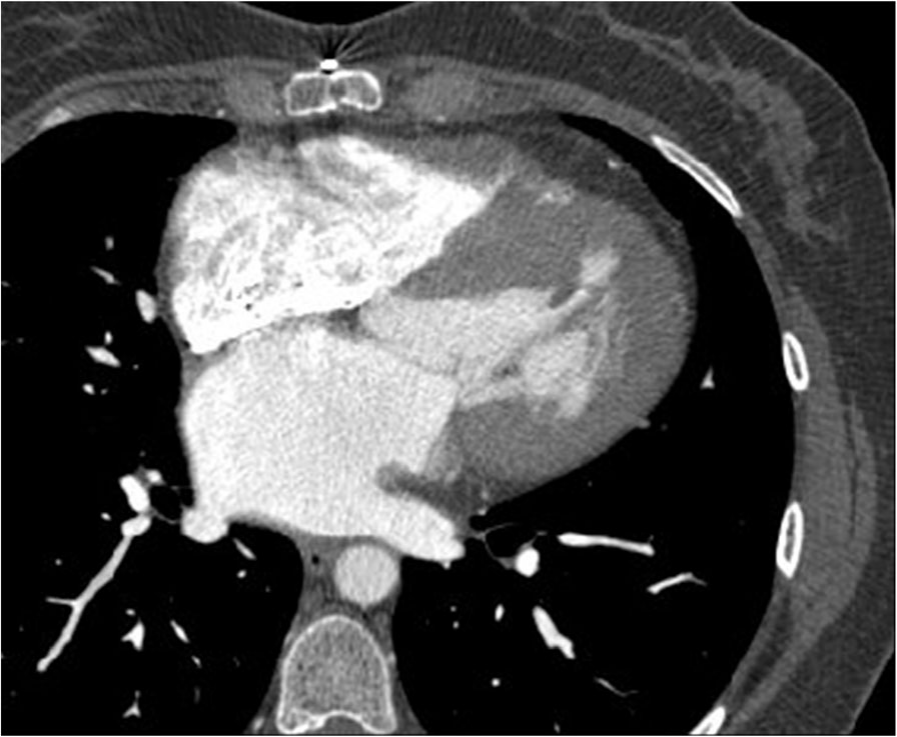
Axial contrast enhanced CT demonstrates the asymmetric form of hypertrophic cardiomyopathy (HCM) on an axial contrast enhanced image (with anteroseptal or mid ventricular thickening) and anatomic narrowing of the left ventricular outflow tract (LVOT) in a 37-year-old female being evaluated for pulmonary embolus
